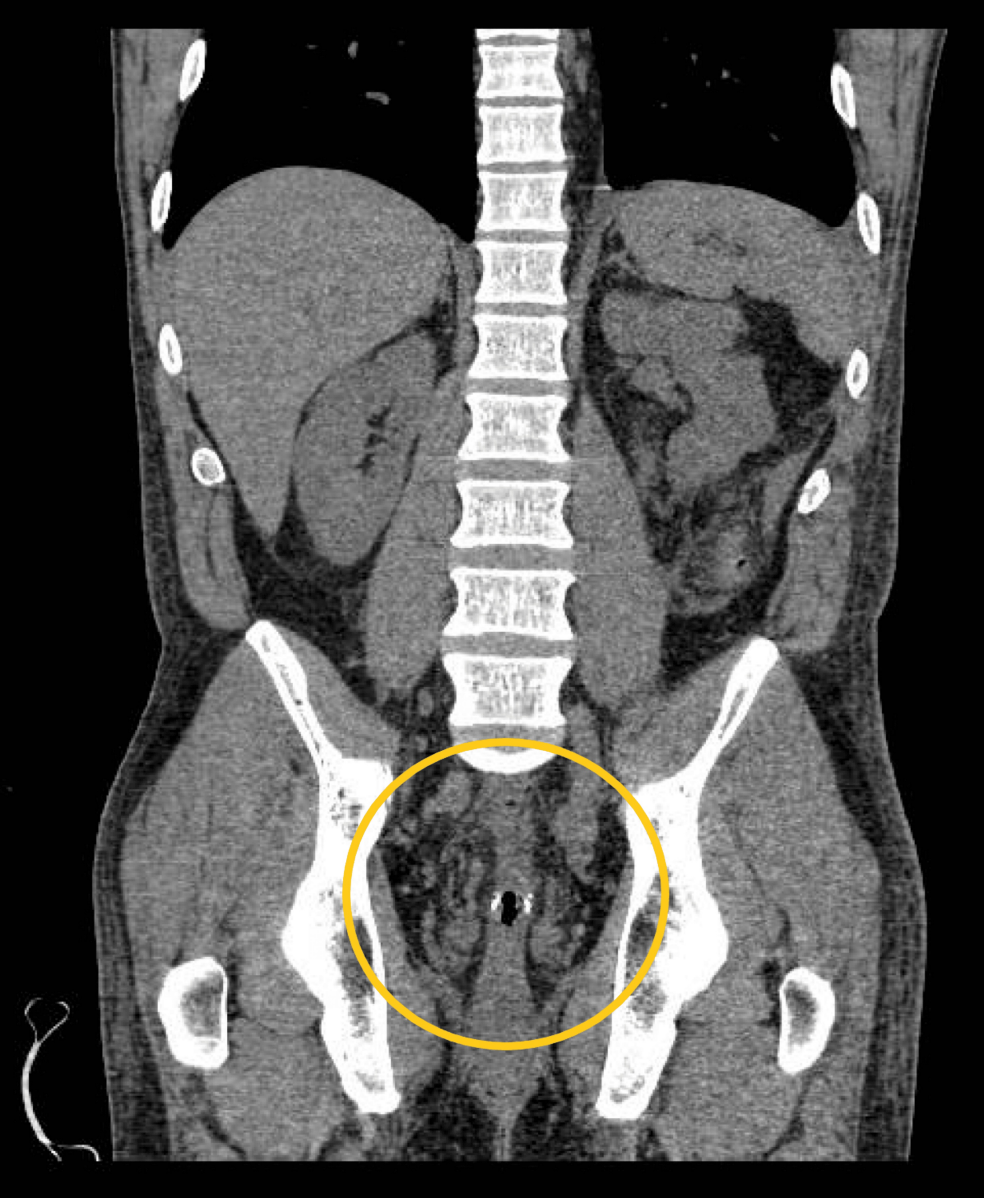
Abdominal computed tomography, coronal plane. Slight thickening of the remaining rectum.
